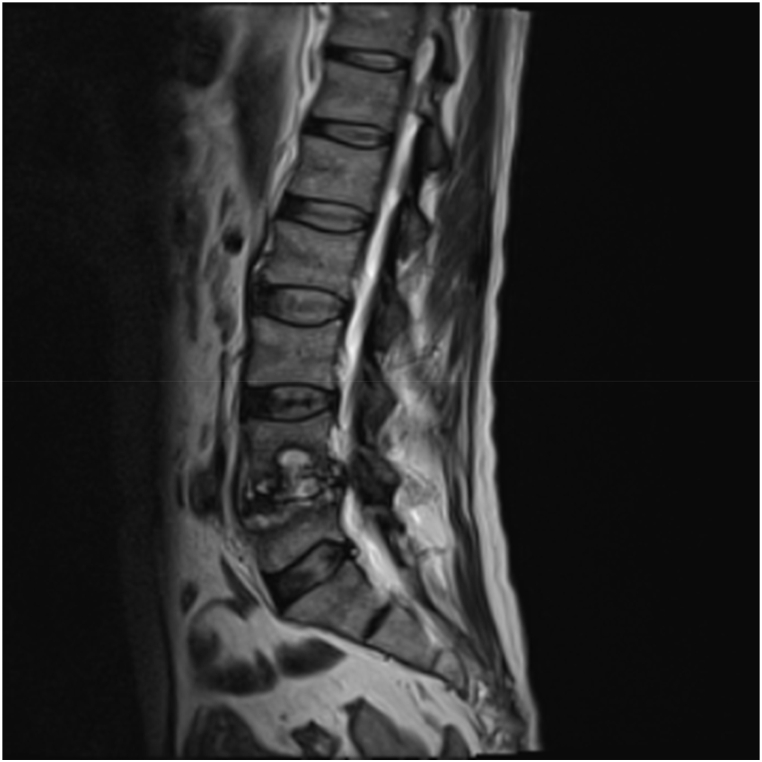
Lumbar Spine Magnetic Resonance Imaging (MRI) showing discitis osteomyelitis at the L4–L5 level without evidence of epidural phlegmon or abscess. No spinal canal stenosis. Also, severe bilateral neural foraminal stenosis at L4–L5.
